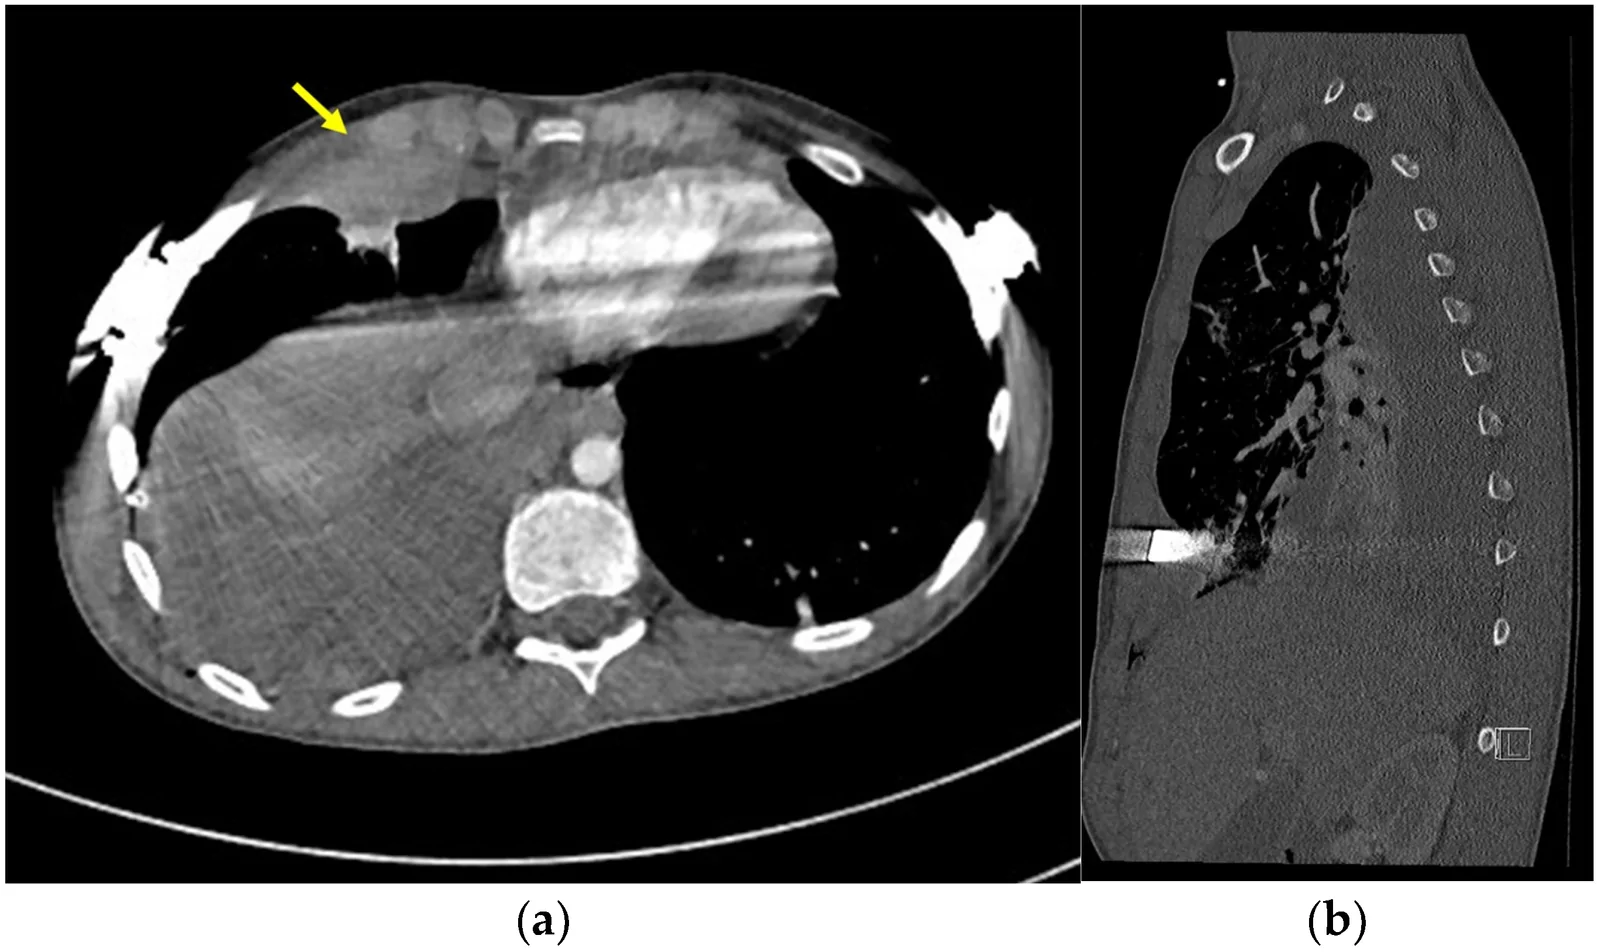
(a,b) CT angiography: suspicion of a relevant lesion to the right-sided internal mammary artery (IMA) adjacent to the pectus bar (yellow arrows).
